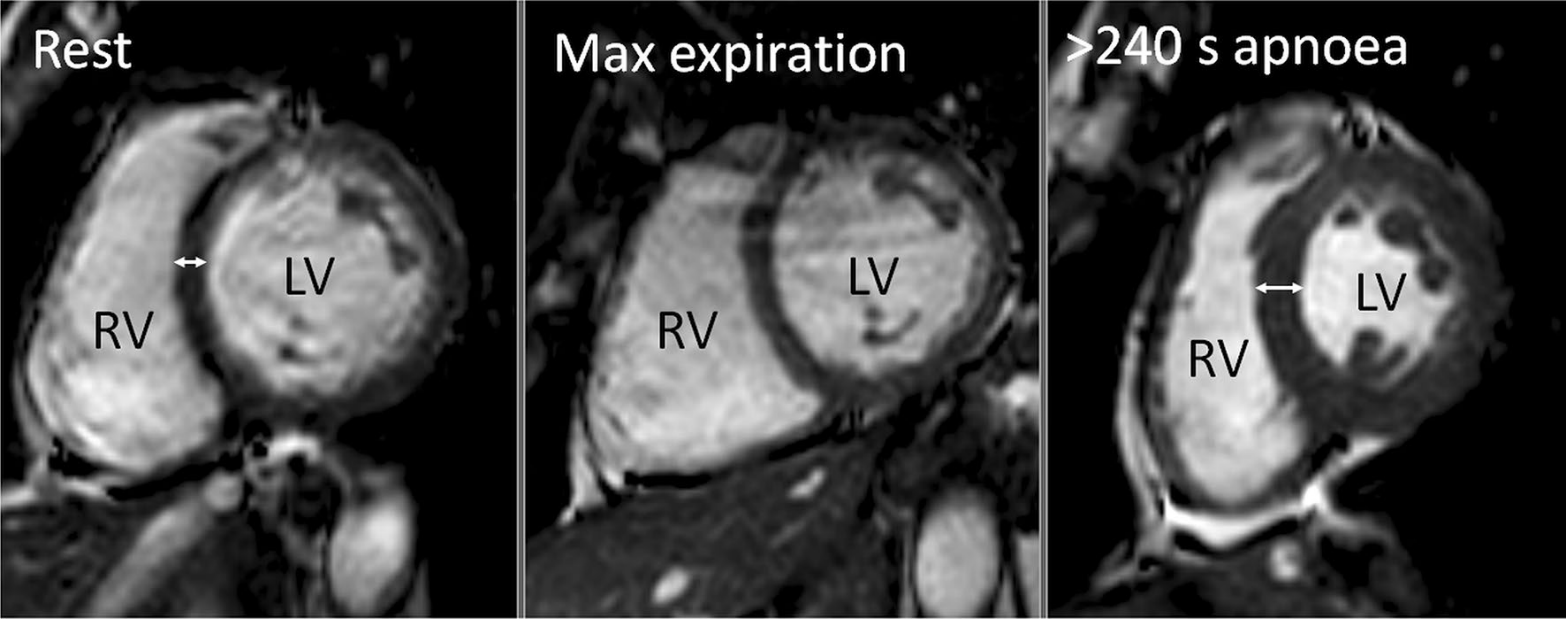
Example of Cardiac MR imaging of one subject, displaying a midventricular short axis view at papillary muscle level at end-diastole. Compared to rest (left panel), the left ventricle (LV) and right ventricle (RV) are similar in size at end of apnea with maximum expiration (center panel). In contrast, at the end of > 240 s of apnea with glossopharyngeal insufflation (right panel) there is a decrease in LV and RV size and thickening of the ventricular wall (double-headed arrows) compared to rest.
